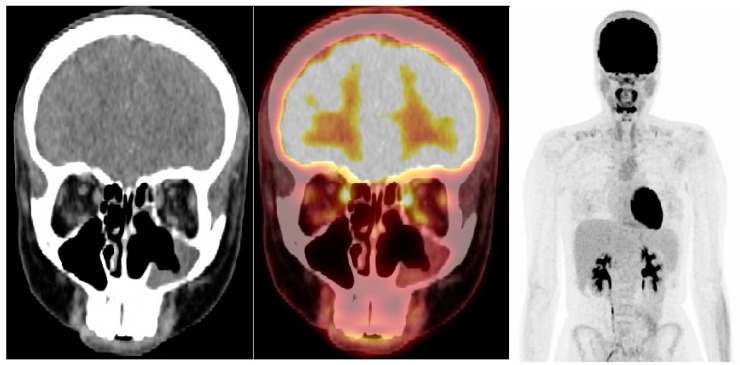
Follow-up scan—(left,middle): CT and fused ^18^F-FDG PET/CT, coronal view; (right): ^18^F-FDG PET, maximal intensity projection. ^18^F-FDG PET/CT 3 months after end of radiation therapy showed no remaining primary tumor or any metastatic disease. There was mildly increased FDG-uptake and mucosal swelling in the upper nasal cavity and frontal sinus, interpreted as an inflammatory reaction to therapy. This case of esthesioneuroblastoma (ENB) is atypical in several aspects. Pediatric cases of ENB are uncommon, as this is a neoplasm most frequently occurring in patients in their 5th and 6th decade [7]. Furthermore, ENBs rarely induce paraneoplastic syndromes and only a few case reports of antidiuretic hormone (ADH) secreting ENBs have been published [8,9,10,11,12,13,14,15,16,17]. Presenting symptoms are usually related to local tumor expansion, such as nasal obstruction and epistaxis [1]. In this case, the disease was diagnosed early due to hyponatremia caused by SIADH, but ENBs are often disseminated at primary staging where cervical lymph node metastases are found in 20–33% of patients. This makes reliable whole-body imaging crucial for these patients. CT is highly valuable in evaluating regional osseous disease, but lacks sensitivity in lymph node metastases and assessment of soft tissue involvement compared to MRI [18]. In a study comparing MRI, ^18^F-FDG PET/CT and endoscopy after primary surgery for ENB in 28 patients, ^18^F-FDG PET/CT was superior to MRI in detecting small lymph node metastases, but did not detect meningeal involvement in three patients which was visualized on MRI [19]. Using ^18^F-FDG PET/MRI in this setting could provide an optimal combination for imaging ENB. Another promising method is somatostatin receptor imaging (SRI) such as ^68^Gallium-DOTATOC PET or ^111^Indium-Octreotide scintigraphy. A subset of ENB expresses somatostatin receptors and the use of SRI in ENB has been described in case reports [20,21,22,23]. Furthermore, radionuclide therapy with somatostatin analogues labeled with beta-emitting isotopes may be a therapeutic option for disseminated ENB [21,22,23]. However, prospective studies are needed to confirm the value of SRI and targeted radionuclide therapy in ENB. In conclusion, ENB is a rare cause of SIADH and other paraneoplastic syndromes, but should be held in mind as the disease may be highly aggressive. Reliable whole-body imaging is important in disease evaluation. As demonstrated in this case, ^18^F-FDG PET/CT can be valuable in staging by excluding or confirming disseminated disease and in follow-up after therapy for ENB.
